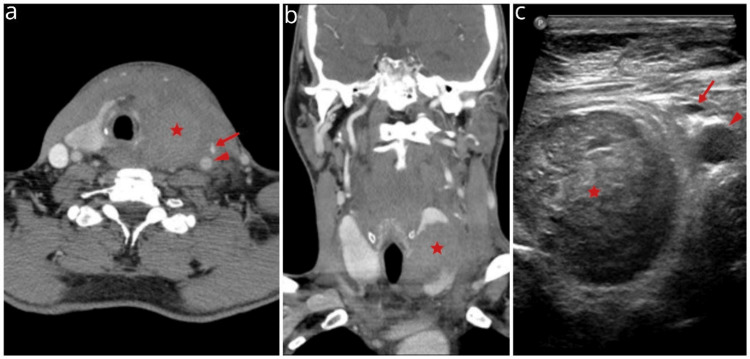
Initial contrast-enhanced computed tomography (CECT) (a, b) and 2D B-mode ultrasound (c) The images demonstrate parenchymal rupture and haematoma (star) of the left thyroid lobe. A narrowed left internal jugular vein (arrow) and displaced left common carotid artery (arrowhead) are also observed.
